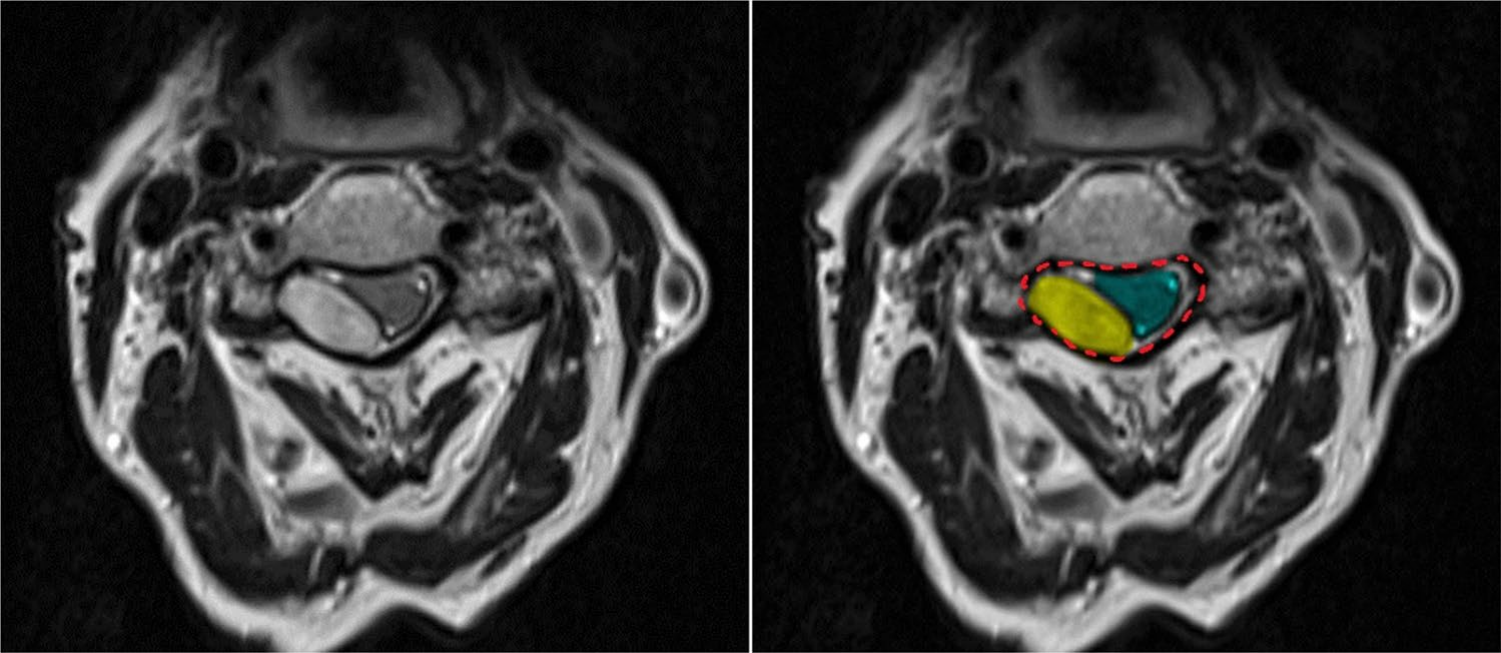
Axial T2-weighted MR image of one of the included patients demonstrating the radiological evaluation. The two images show the same axial slice. In the right image the spinal canal is outlined in a red dashed line, the spinal cord is highlighted in green, the SSEH is highlighted in yellow. The highlighted axial areas’ surfaces in mm^2^ were determined with the SECTRA IDS7 software
